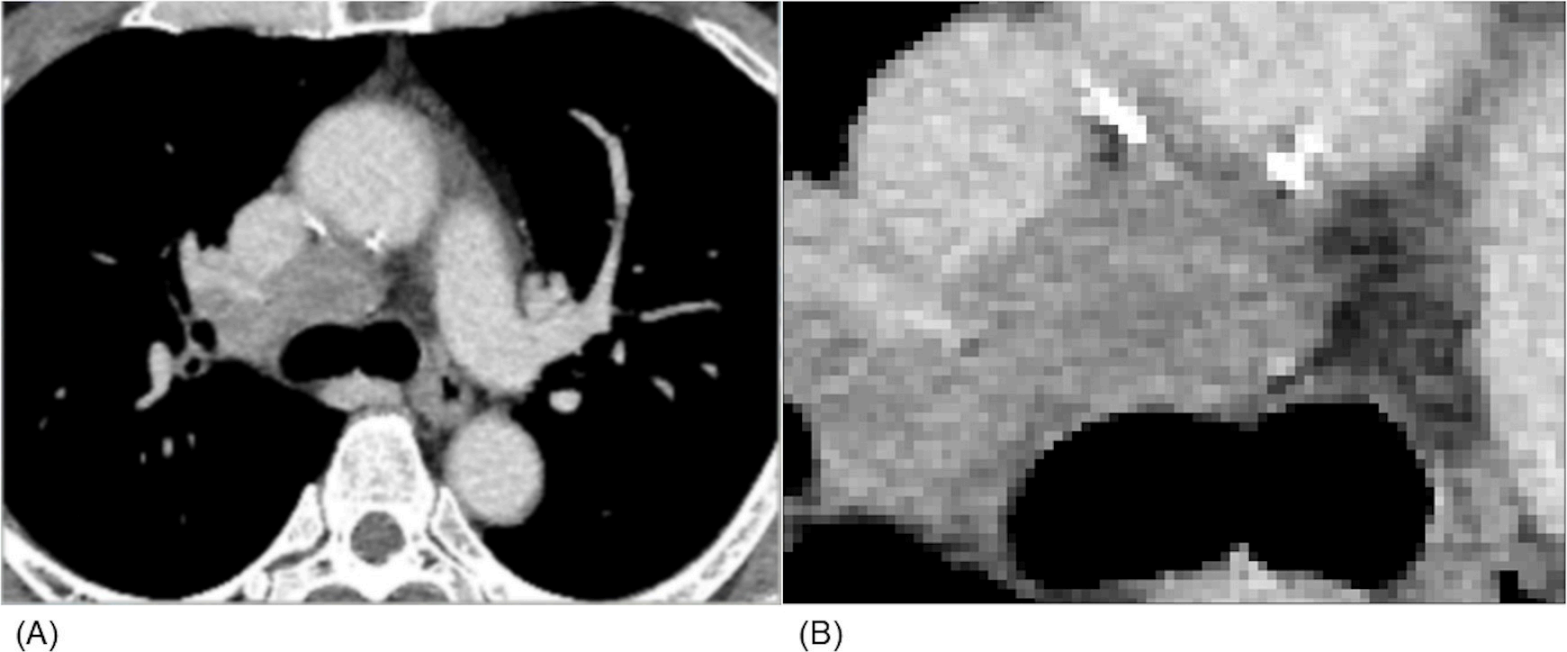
CT image of lymph node metastasis from small cell lung cancer. A large lymph node, which was proven by a transbronchial needle aspirate to be a lymph node metastasis of small cell lung cancer, is depicted on an enhanced CT image (A). Density and contrast enhancement of the node appears slightly heterogeneous; however, massive necrosis is not observed (B).
